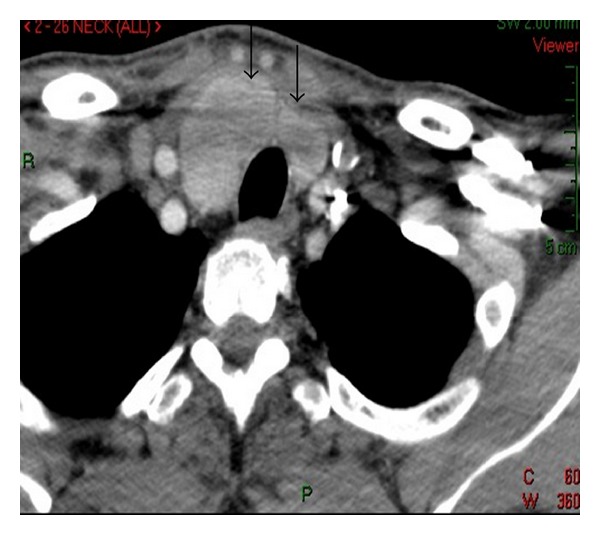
Patient's CT imaging showing a remarkable inflammatory process on the posterior portion of the neck and upper back. Note the accidental findings of diffusive enlargement of right lobe and isthmus of thyroid gland (see two black arrows) related to unrecognized subclinical goiter.
